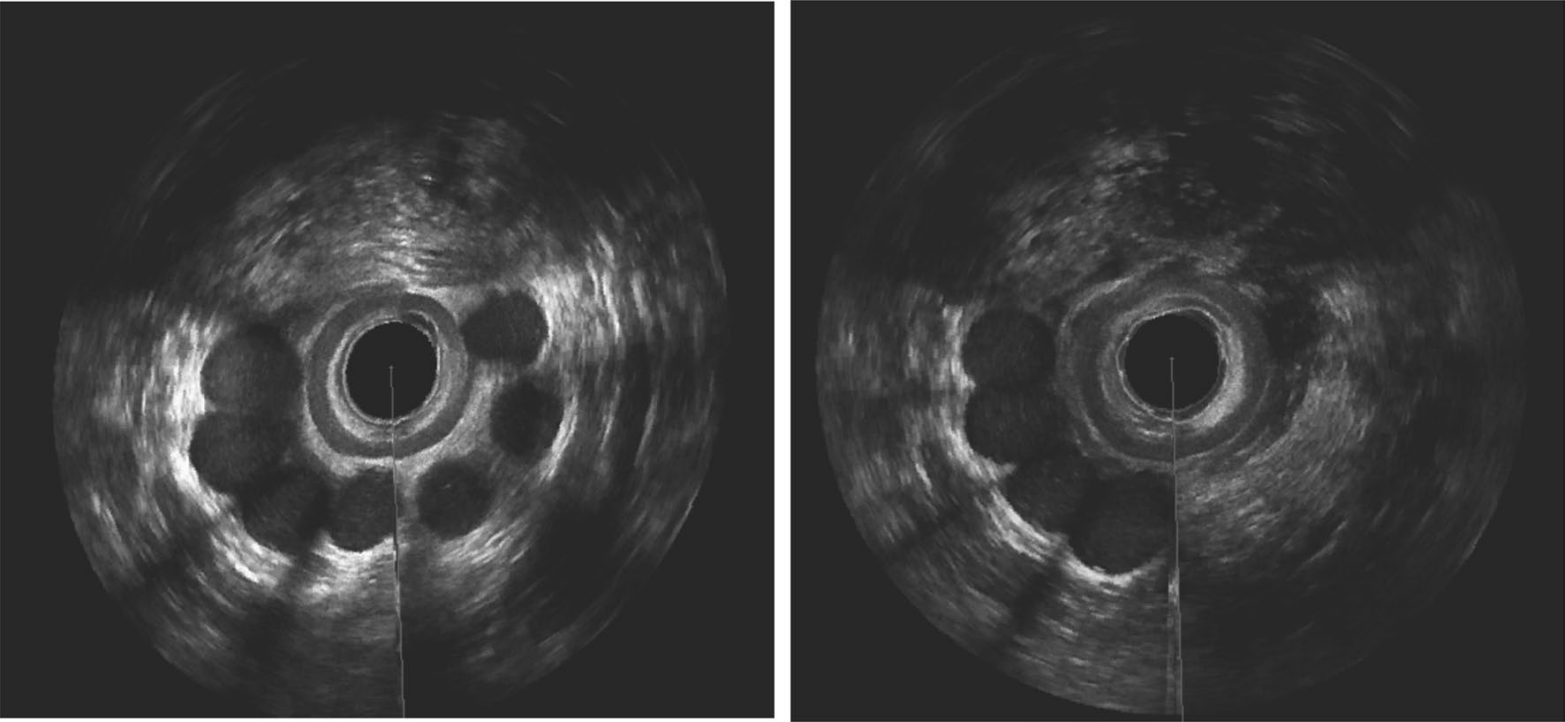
A 3D anal Endosonography 1 month postoperatively: 7 prostheses in the intersphincteric space in correct postion. B 3D anal Endosonography 12 months postoperatively: 4 prostheses in the intersphincteric space in correct position, the other 3 prostheses are not seen in same place as before
